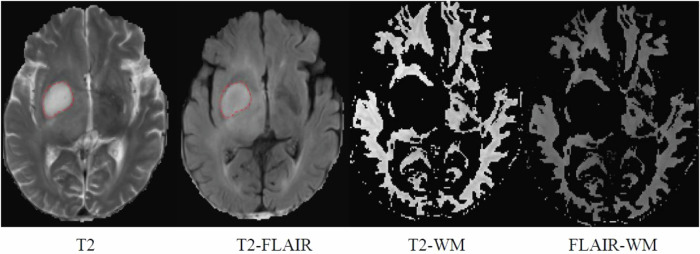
Quantification of the T2-FLAIR mismatch ratio. WM, white matter
